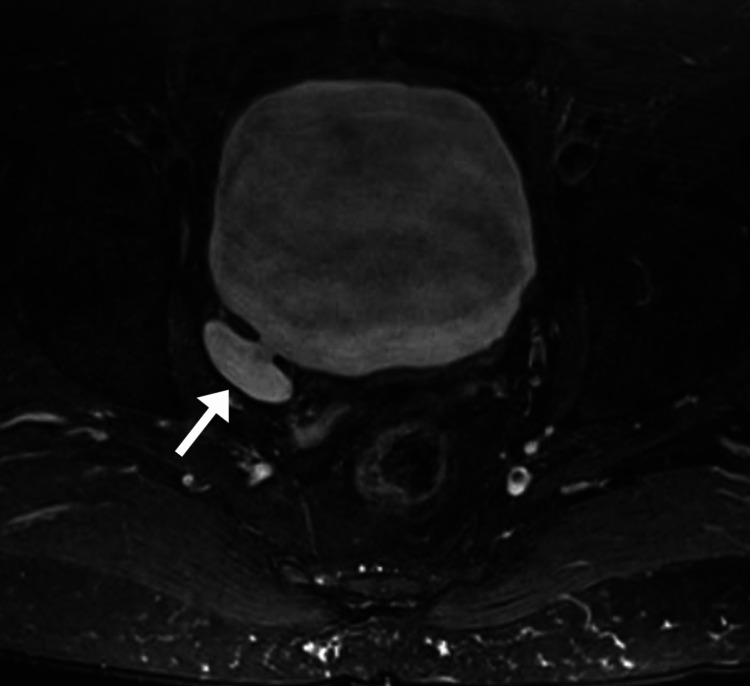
Axial lumbosacral plexus MRI. This T2 sequence oriented through the mid-pelvis shows the bladder measuring 9.4 cm x 8.9 cm with a small diverticulum (arrow) which was deemed to be incidental by the neurologist and urologist.
